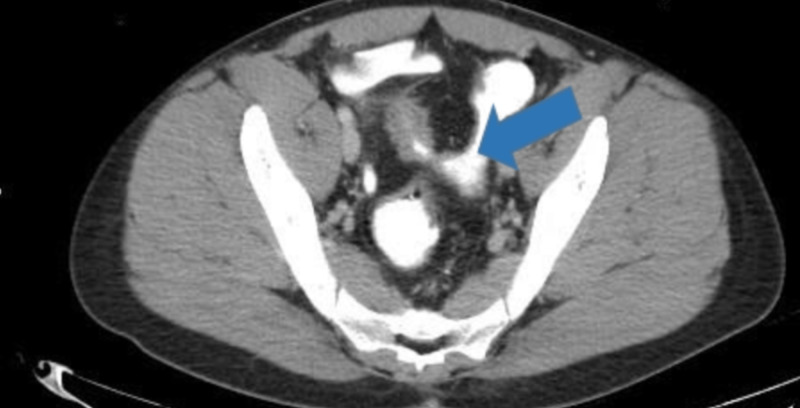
CT scan showing distal ileum with pseudodiverticulum thickened wall indicated by the blue arrow
